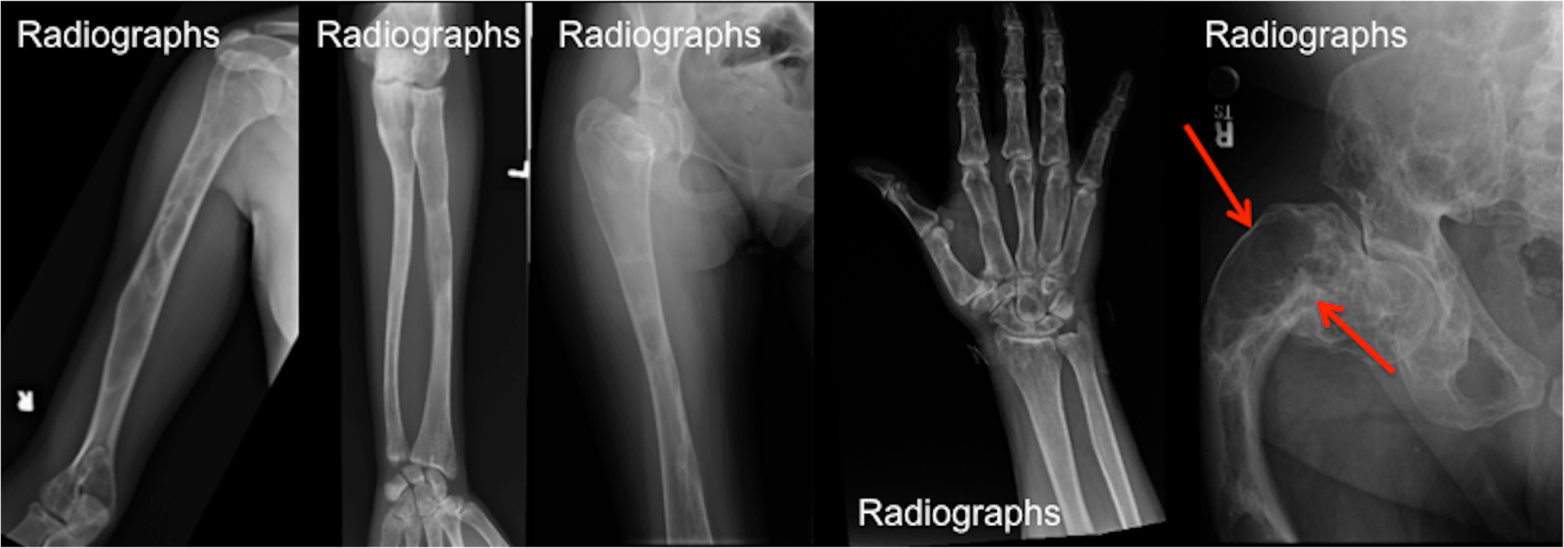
The radiographic appearance of fibrous dysplasia (FD) and the rind sign. a–e Frontal radiographs demonstrate classic FD lesions in appendicular skeleton. A classic lucent lesion surrounded by a layer of sclerotic reactive bone (so-called the rind sign). The rind sign is most commonly seen in the proximal femur (*red arrow*)
